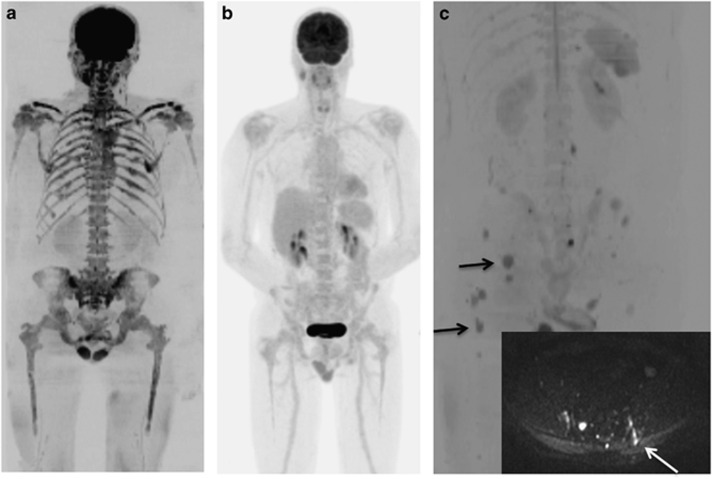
WB-DWI improves detection of diffuse marrow infiltration compared with FDG PET–CT and detects trephine sampling error. WB-DWI b900 maximum intensity projection image (a) and FDG PET–CT (b) in the same patient. WB-DWI demonstrates diffuse infiltration of the bone marrow; FDG PET–CT shows no evidence of disease. WB-DWI b900 maximum intensity projection image (c) demonstrates multifocal disease (examples shown by arrows). Axial b900 image (superimposed) shows the trephine tract (arrow), which does not sample a focal lesion.
